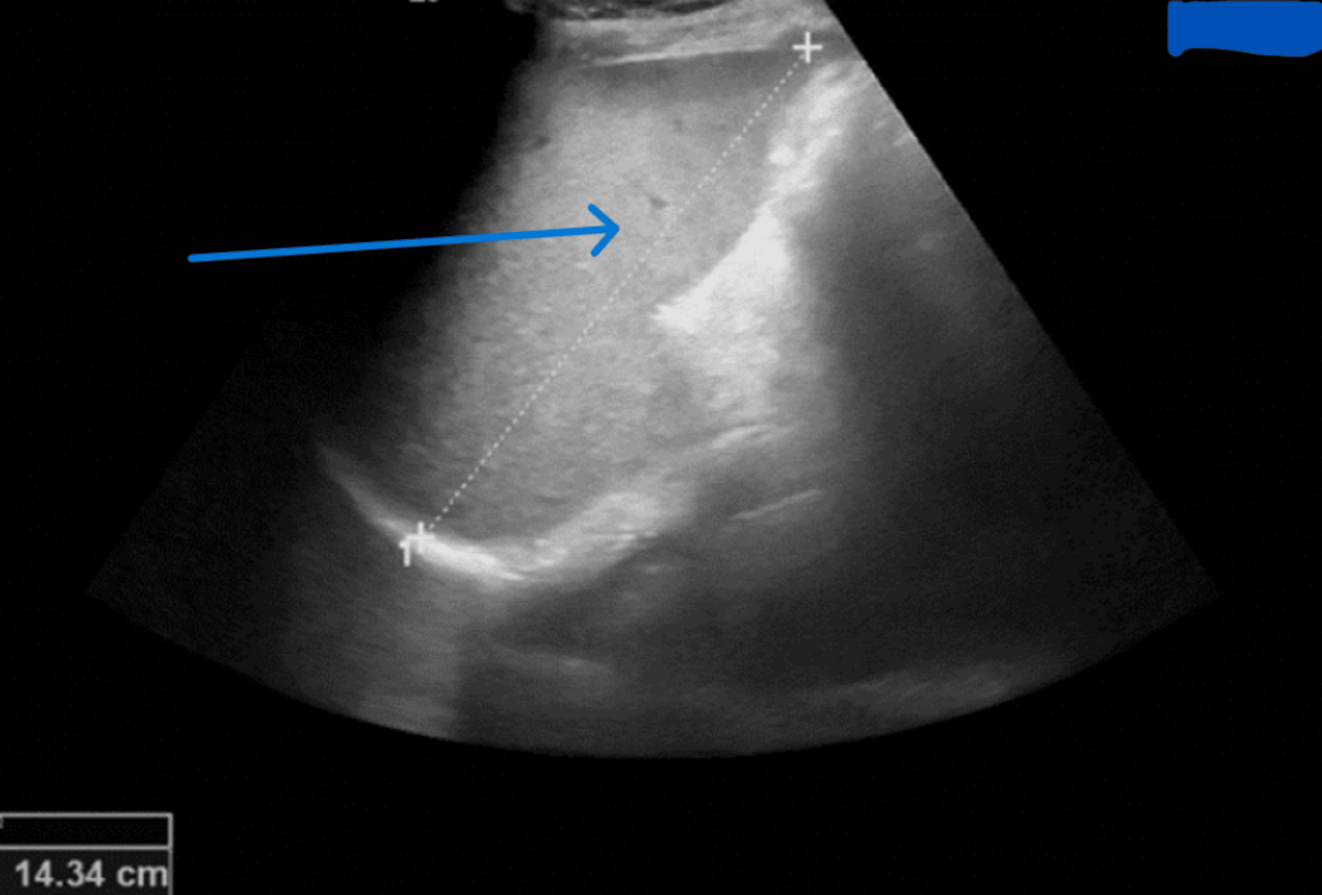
Ultrasound scan showing splenomegaly (blue arrow).
